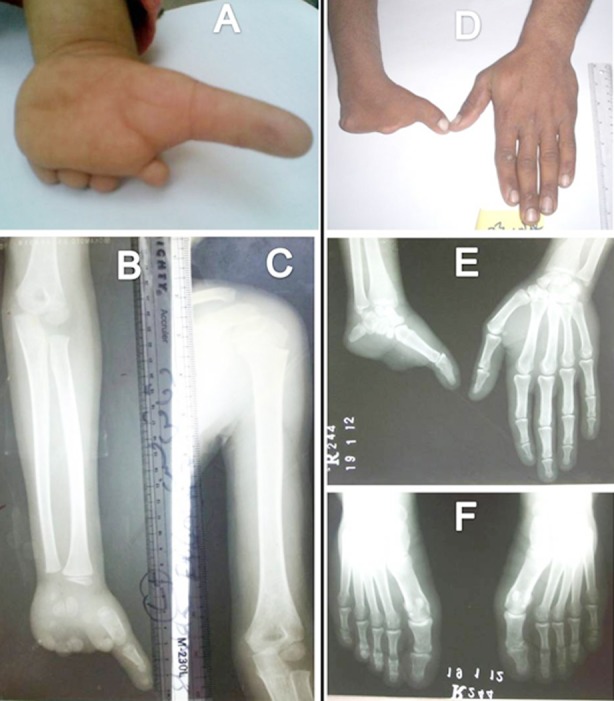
(A-C): Photographs and roentgenographs of individual I. (D-F): Phenotype in individual II.
